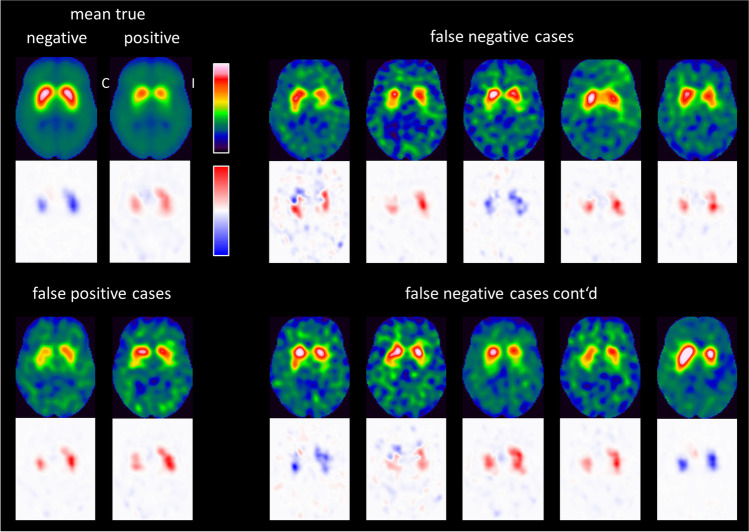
Individual relevance maps of the 12 amongst the 288 test cases that were misclassified by the CNN. The mean DAT-SPECT and the mean relevance map in true negative and true positive cases (from Fig. 3) are shown for comparison. (I / C, Ipsilateral / Contralateral to the hemisphere with lower specific FP-CIT binding ratio in the putamen)
